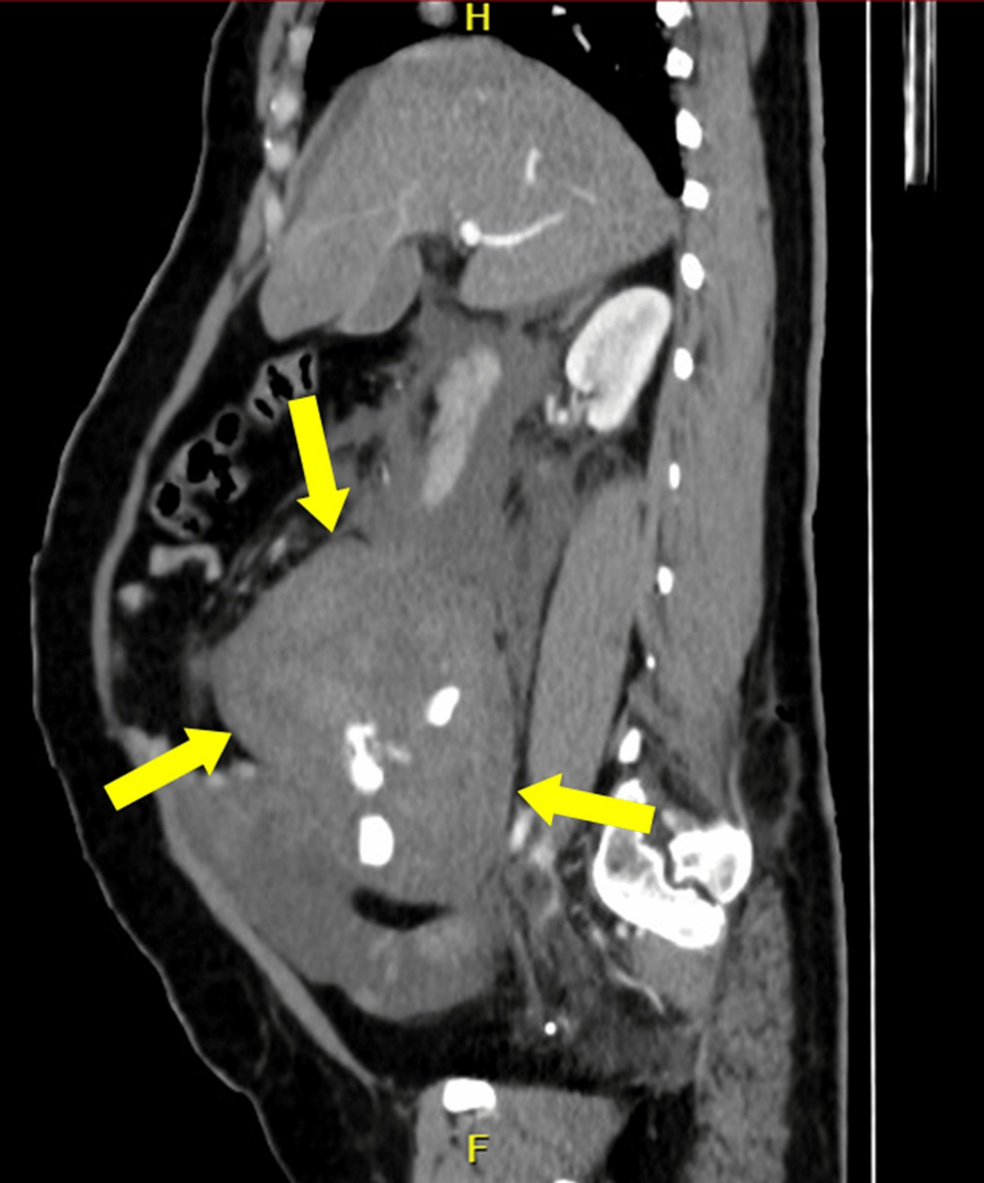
Vascular extravasation and large retroperitoneal hematoma on CTA (sagittal view). CTA: computed tomography angiography
